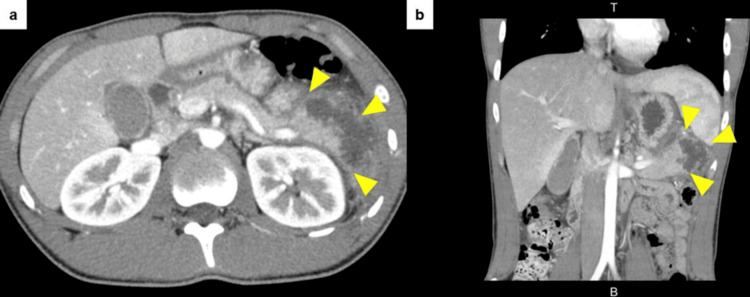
Follow-up CT scan on day 8 of hospitalization. A 4-cm cyst is found in the pancreatic tail. (a) Horizontal section image. (b) Coronal section image. Arrowheads show the extent of the cyst.
